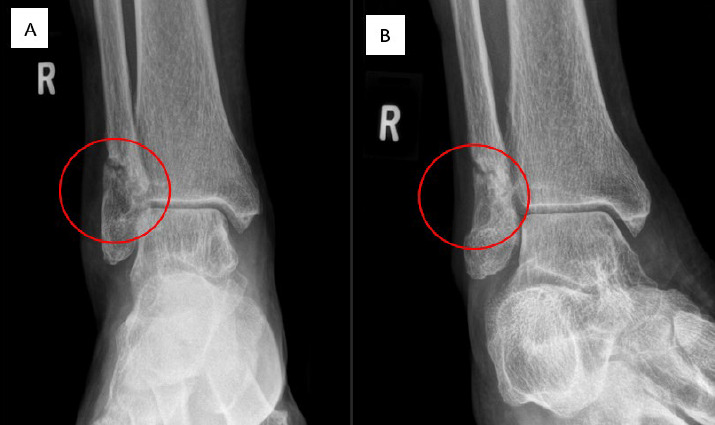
Repeat right ankle radiographs in AP (A) and mortise (B) views after 6 months of treatment showing a more well-defined lucent lesion with a sclerotic margin at the distal fibula.
